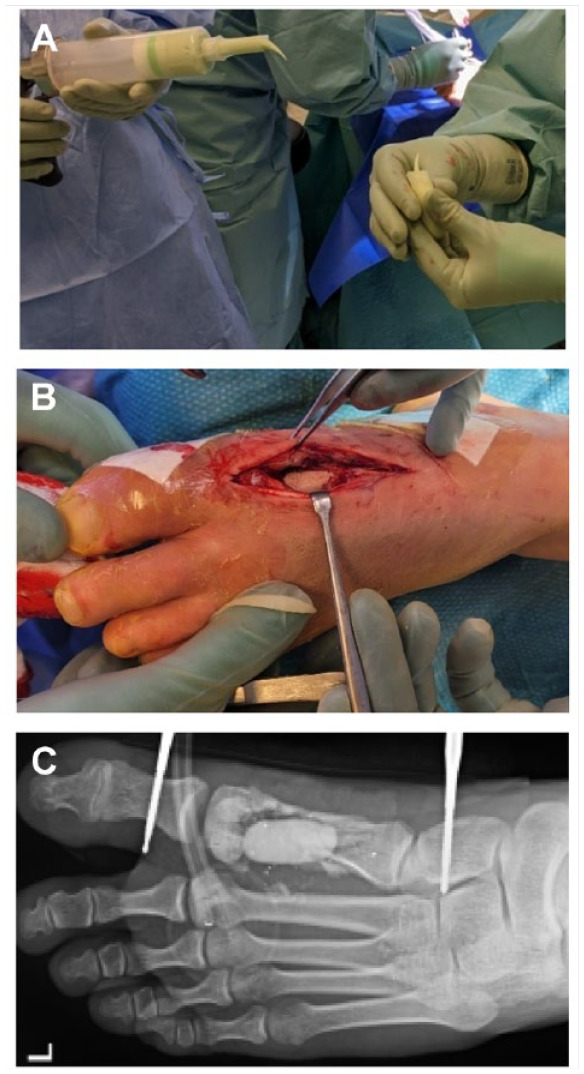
The cement spacer was prepared (2A), and installed into the bony defect (2B). The radiograph shows the cement spacer in the region of the first metatarsal bone (2C).
